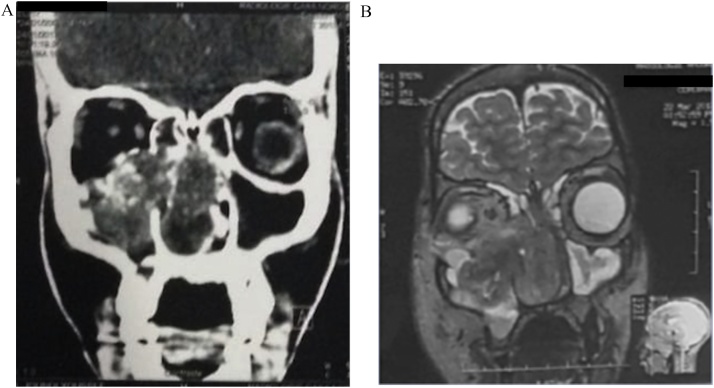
Imaging findings in CE-CT and MRI. (A) CT showed heteregenous tumor mesuring 8.7 × 6.5 cm, heterogenously enhancing with important lysing of the inner wall of the right orbit. (B) MRI showed confirmed high vascularized and extensive mass filling the right nasal cavity.
